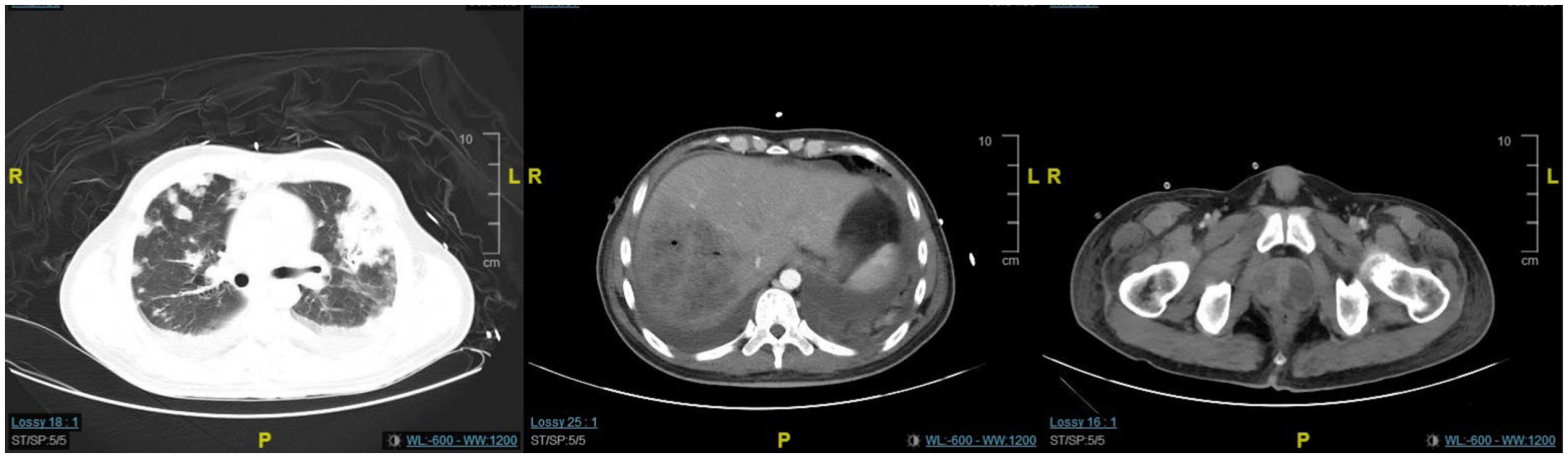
The CT scan revealed minimal improvement in the lung and prostate lesions, while the liver abscess showed significant resolution.
